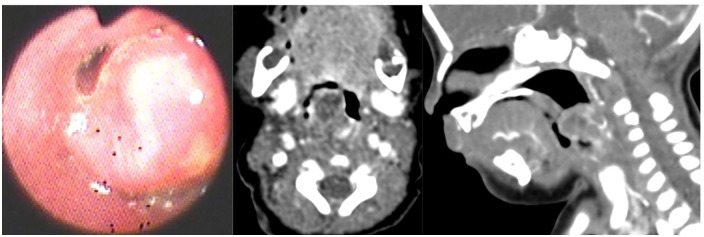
A 19-hour-old female. (A) Fiberoptic laryngoscopy showed a large globular mass (white arrow) with a smooth mucosal surface in the posterior pharyngeal wall. CT revealed a 1.4-cm sized cystic lesion in the posterior pharyngeal wall (red circle): (B) axial contrast-enhanced CT; (C) sagittal contrast-enhanced CT.
